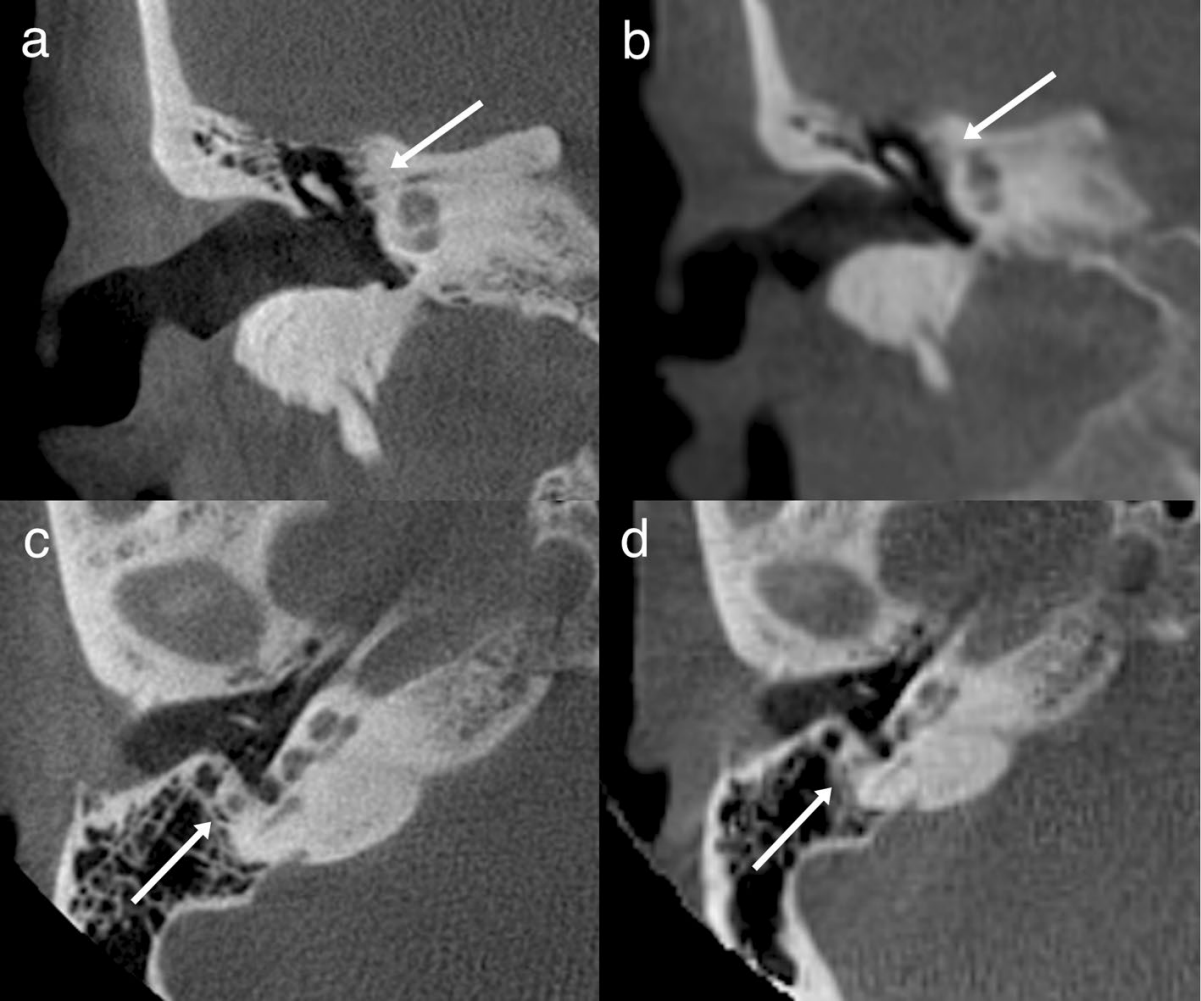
Two example images from the same patient: panels a and c were taken with HR CBCT and panels b and d with ULD CBCT. Both scans were rated as high quality. Panels a and b are in the coronal plane, and panels c and d are in the axial plane. The course of the facial nerve is visible
